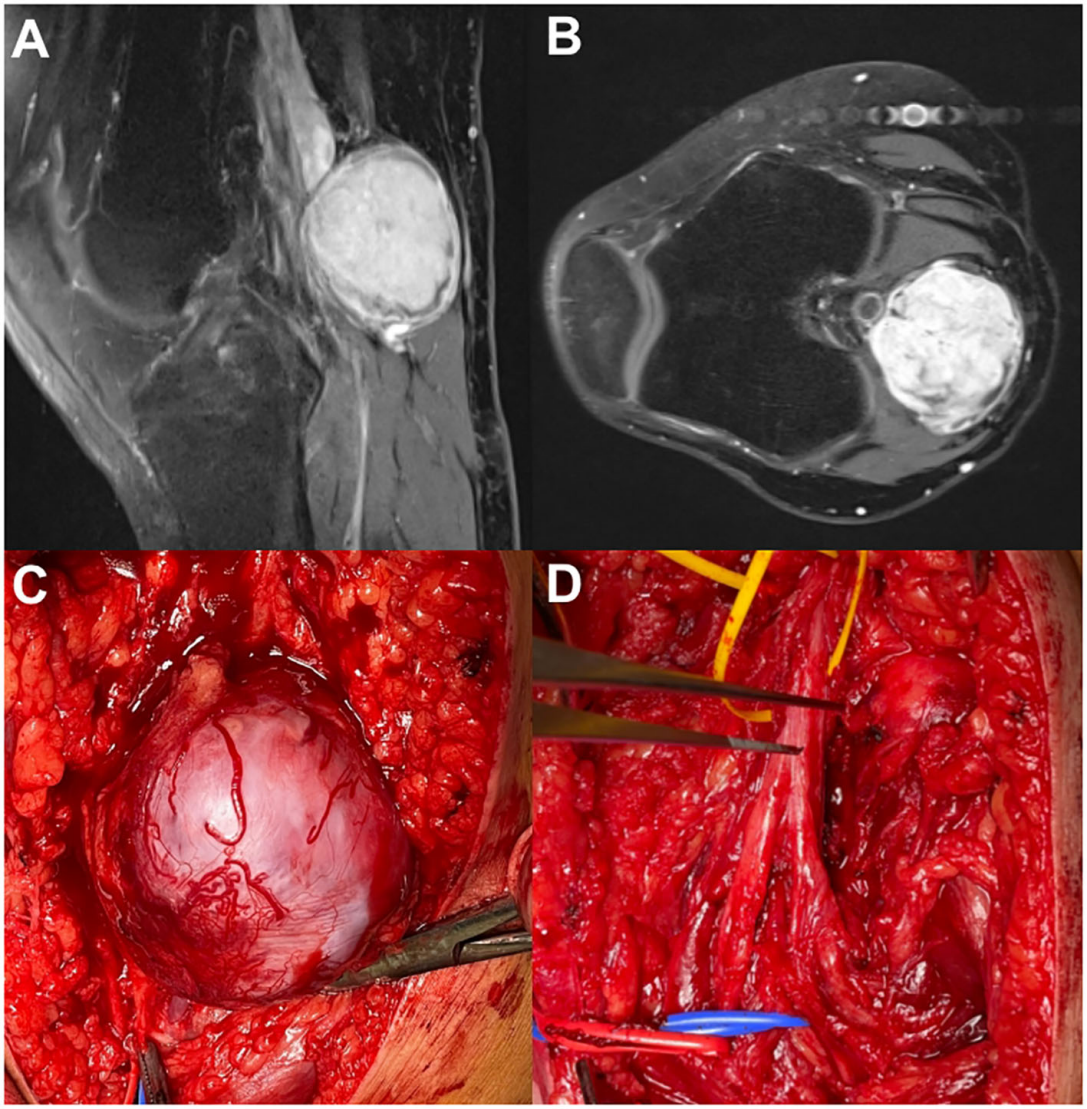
NTRK rearranged spindle cell neoplasm. (A) MRI—sagittal view. (B) MRI—axial view. (C) Open exploration (popliteal approach) of the tumor and tibial nerve. (D) Tibial nerve after tumor removal.
